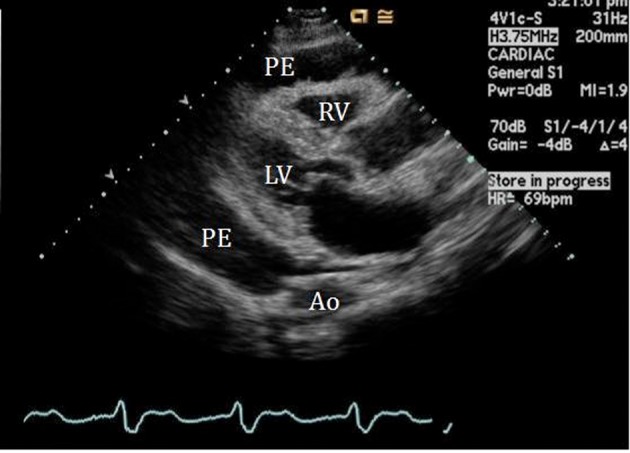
2-D Parasternal long axis view showing large pericardial effusion (PE) surrounding the right (RV) and left ventricle (LV).
